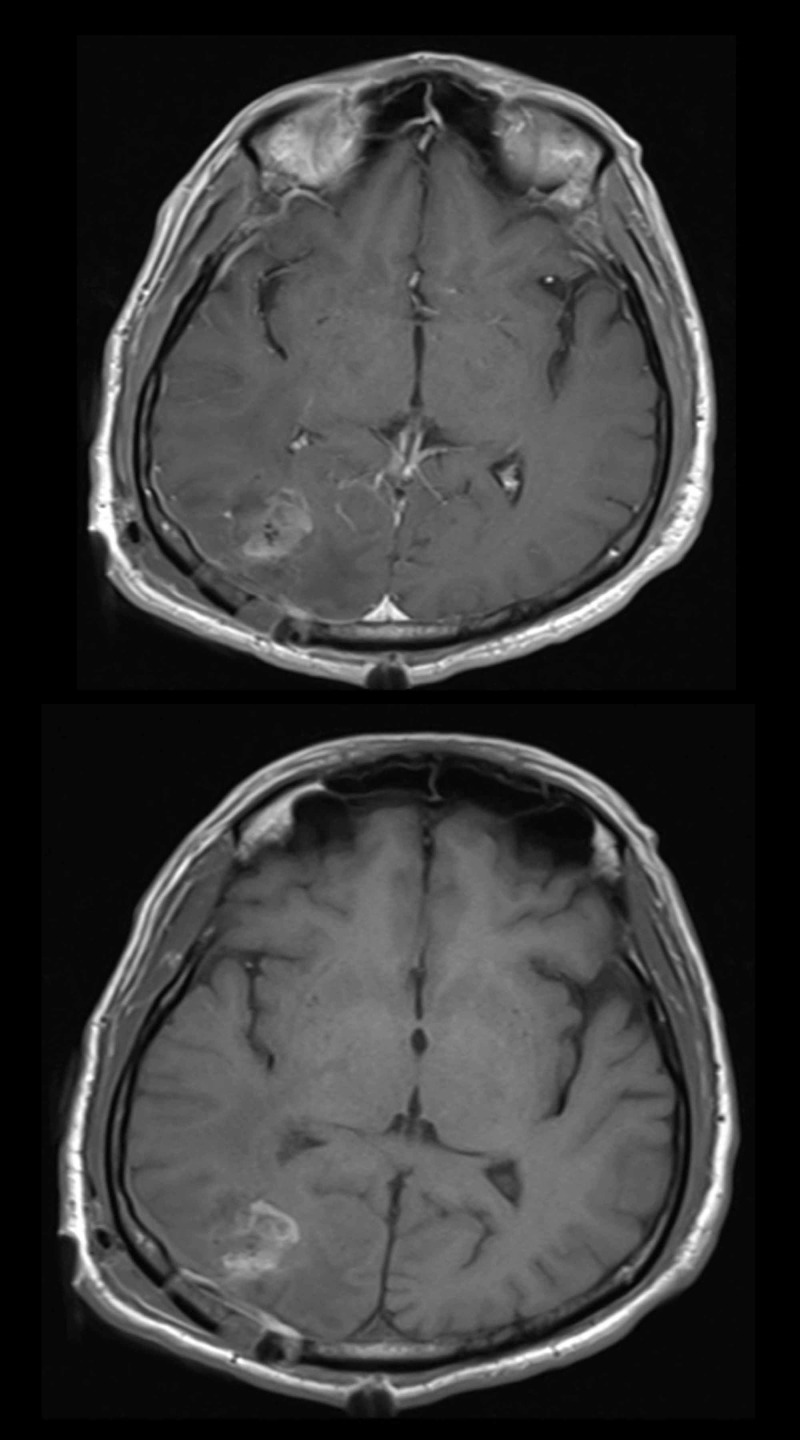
Follow up MRI T1 sequence with contrast (upper) and T1 sequence without contrast (lower) at 12 months showing no evidence of disease
